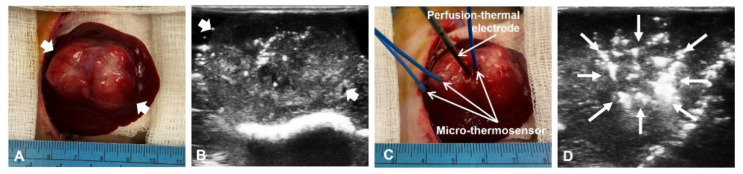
In vivo experimental set up. (A) A hepatic VX2 tumor (between arrows) in a living rabbit is successfully created and detected with ultrasound imaging (B). (C) The 3D multi-modal electrode is inserted into the tumor, while three micro-thermosensors positioned at different points across the tumor, including the center (the electrode core), the middle (between the center and tumor margin), and at the tumor margin. (D) The intratumoral positioning of the electrode, as well as deploying the appropriate array of its prong needles (arrows) are precisely monitored and controlled under real-time ultrasound imaging guidance.
